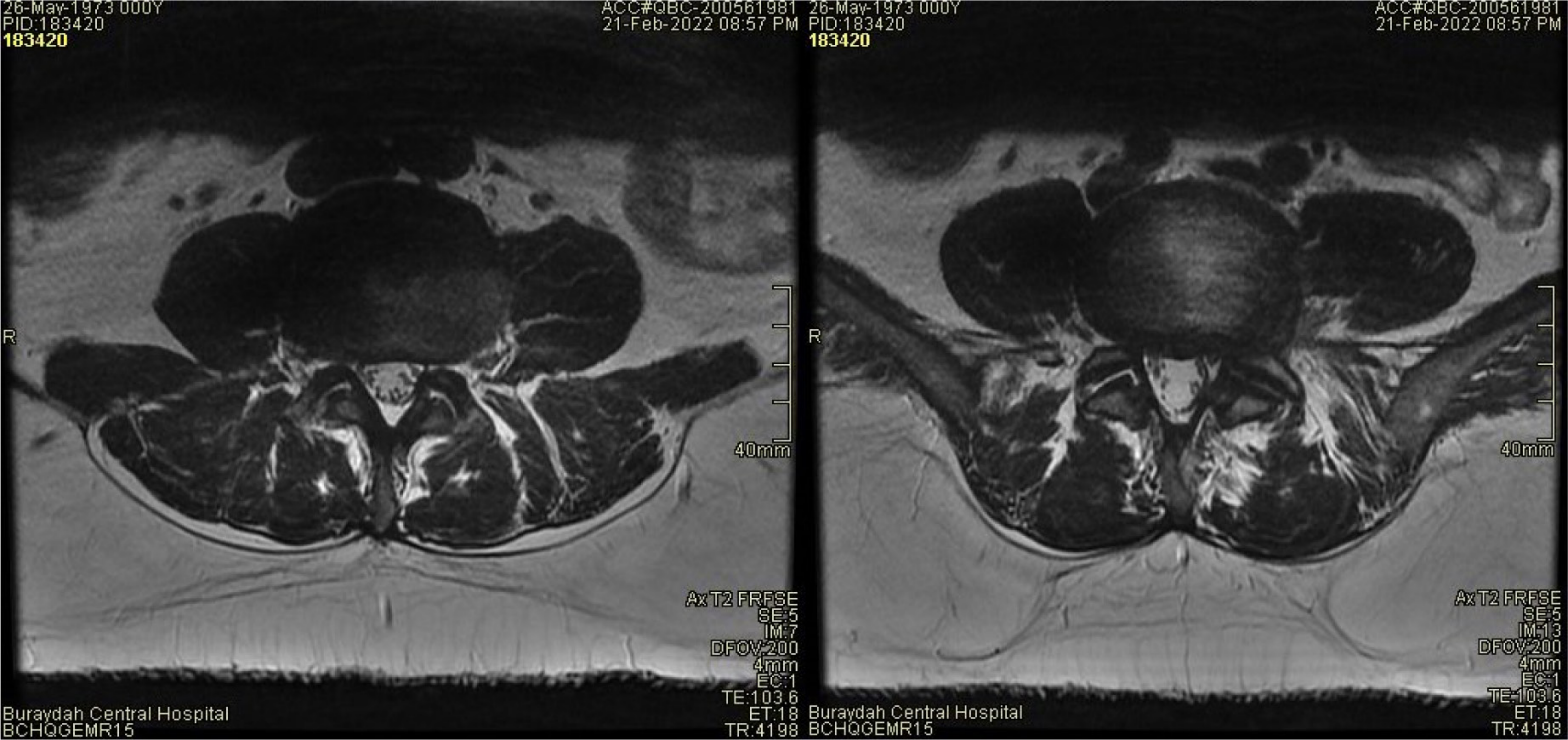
Axial MRI after treatment showing reduced bulging of discs between L5 and L3
